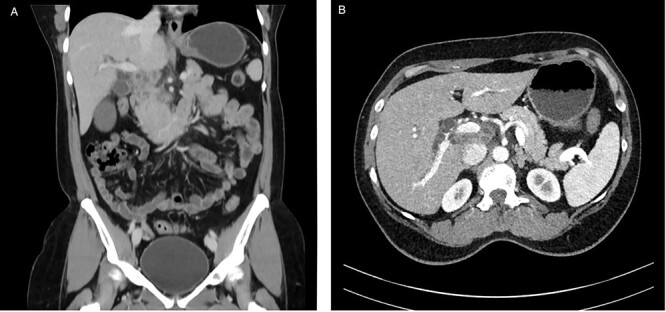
CT with contrast (A) coronal view and (B) axial view: cystic solid mass in the region of the hepatic hilus with unclear association to the head of the pancreas.
